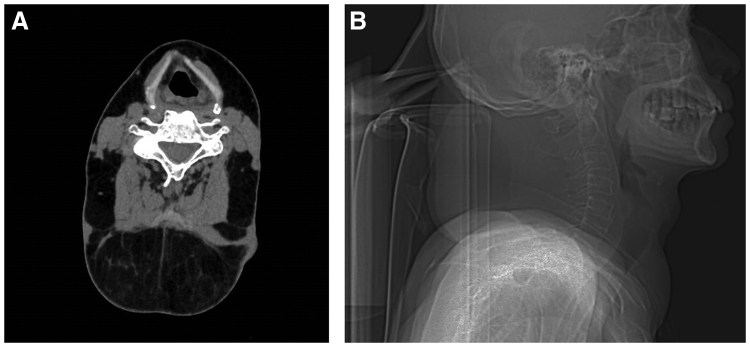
Imaging studies of the neck. (A) Axial computed tomography (CT) scan showing symmetrical, non-encapsulated adipose tissue proliferation in the neck; (B) plain radiograph of the neck showing soft tissue thickening without bony abnormalities.
